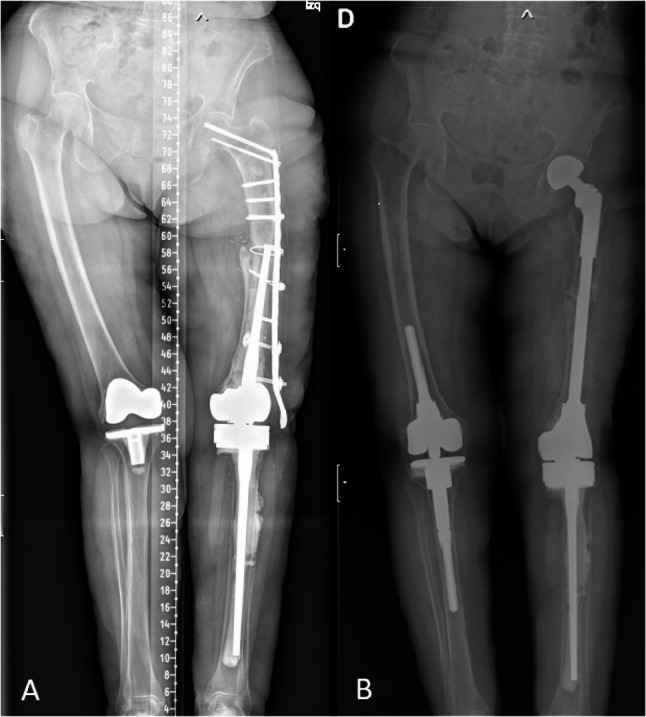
A Preoperative anteroposterior radiograph demonstrating failure of fixation of a periprosthetic femoral fracture secondary to femoral nonunion in an elderly female patient. Preoperative cultures were negative. B Postoperative radiograph showing one-stage reconstruction with removal of previous hardware and implantation of a total femoral replacement using a push-through fixation system. Intraoperative cultures subsequently yielded Staphylococcus epidermidis
